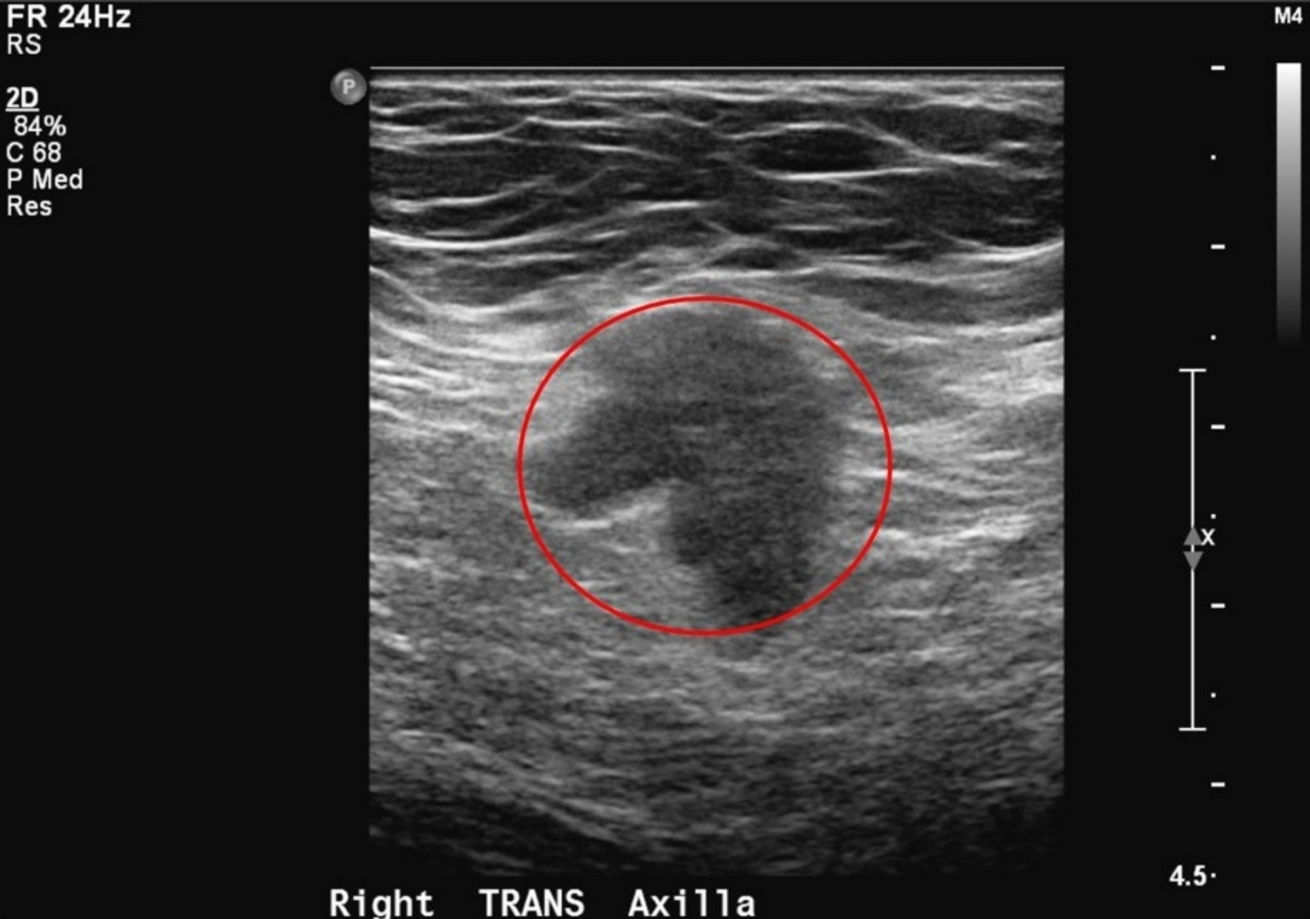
Right axilla diagnostic ultrasound Morphologically abnormal axillary lymph node measuring 19 mm (red circle).
